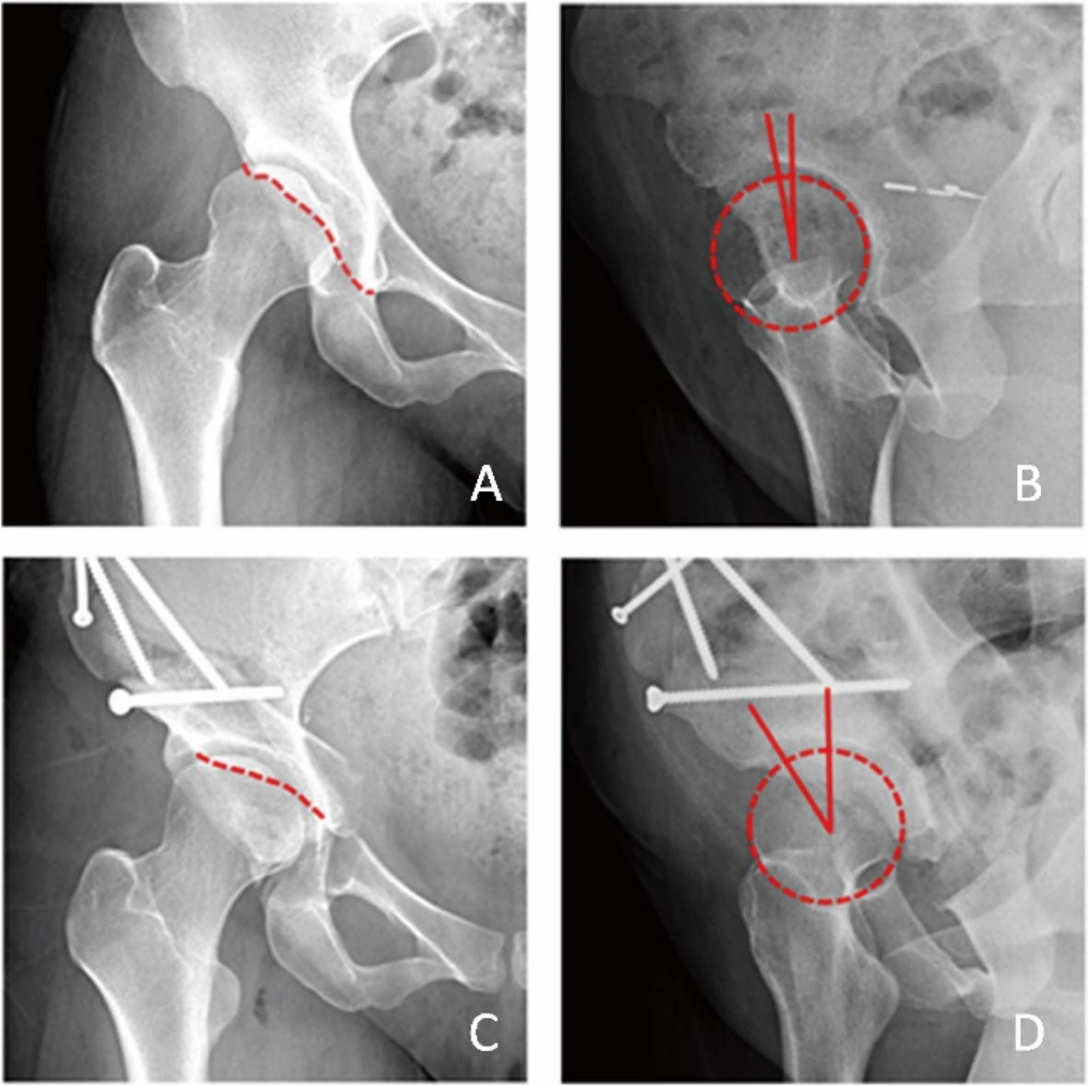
A patient with hip dysplasia. A. Preoperative pelvic AP radiograph of the anterior rim of the acetabulum with a normal appearance; the lateral rim of the AIIS was visible anterolateral to the anterior rim line of the acetabulum. B. Preoperative FP radiograph showing deficient anterior coverage of the acetabulum. C. Postoperative pelvic AP radiograph showing no obvious changes in the anterior rim of the acetabulum. D. Postoperative FP radiograph showing normal anterior coverage
